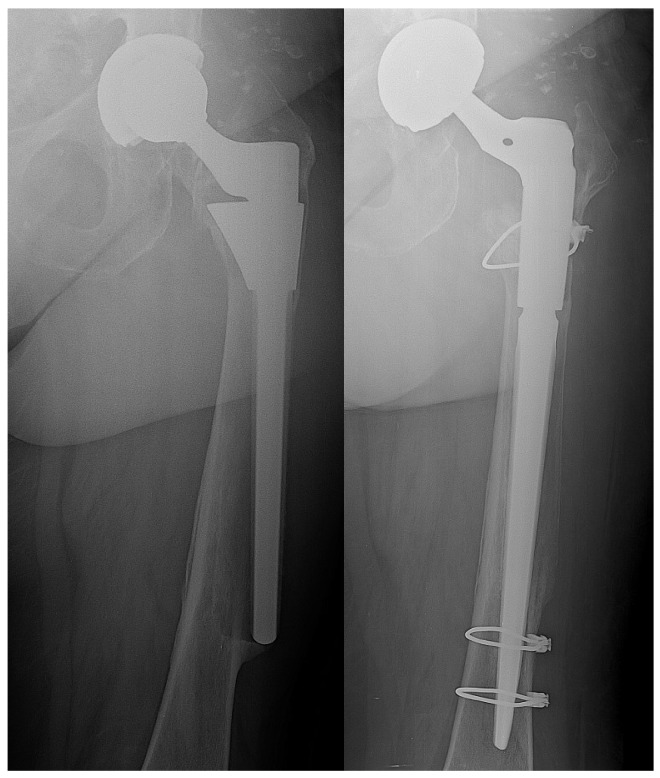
Aseptic loosening of femoral stem—stem and mobile components revision with hydrogel antibacterial coating. On the left side preoperative X-ray; on the right side five months postoperative follow-up.
